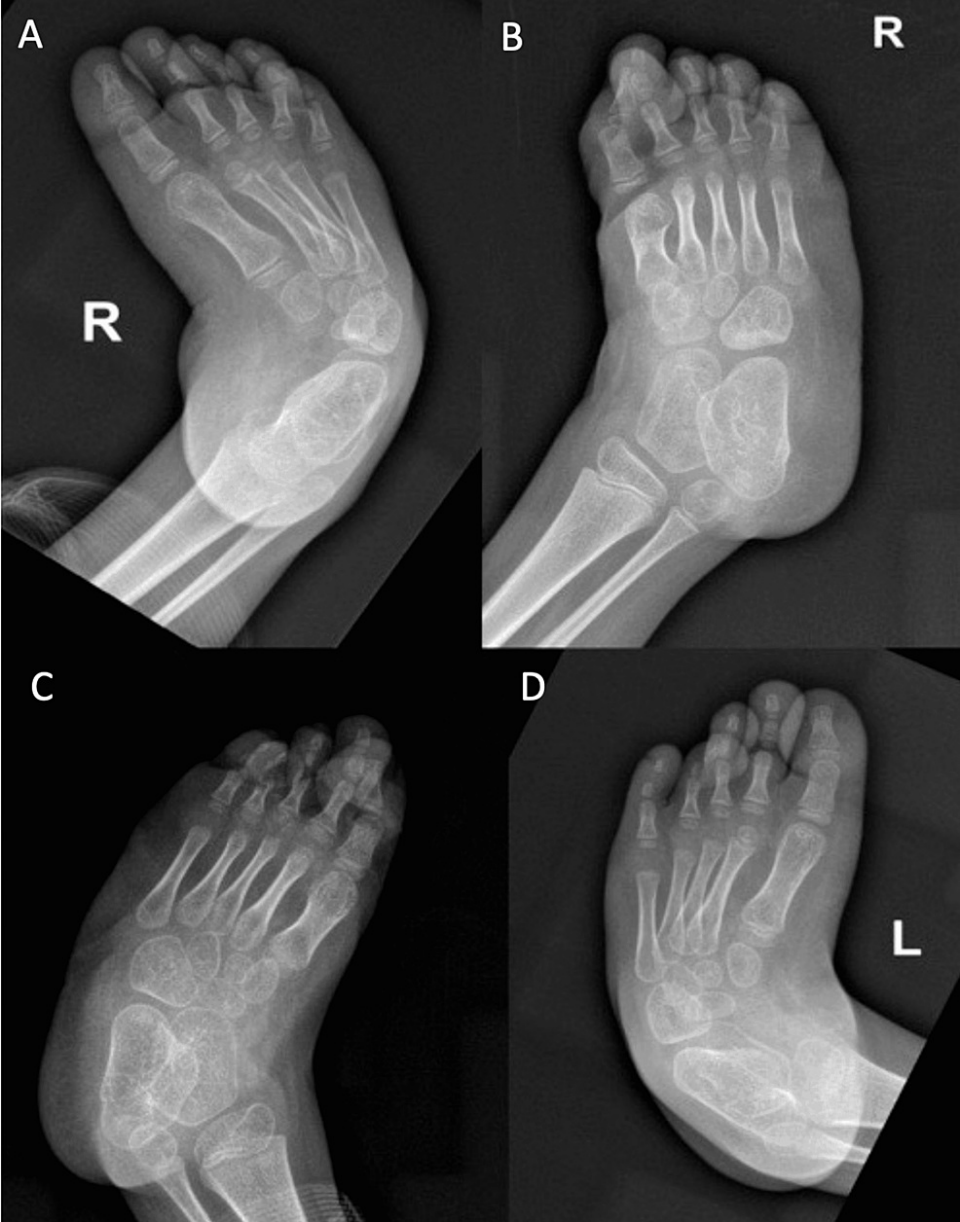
Preoperative x-ray images of Case 2 (A) Anteroposterior (AP) view of the right foot preoperatively. (B) Oblique view of the right foot preoperatively. (C) Oblique view of the left foot preoperatively. (D) AP view of the left foot preoperatively.
